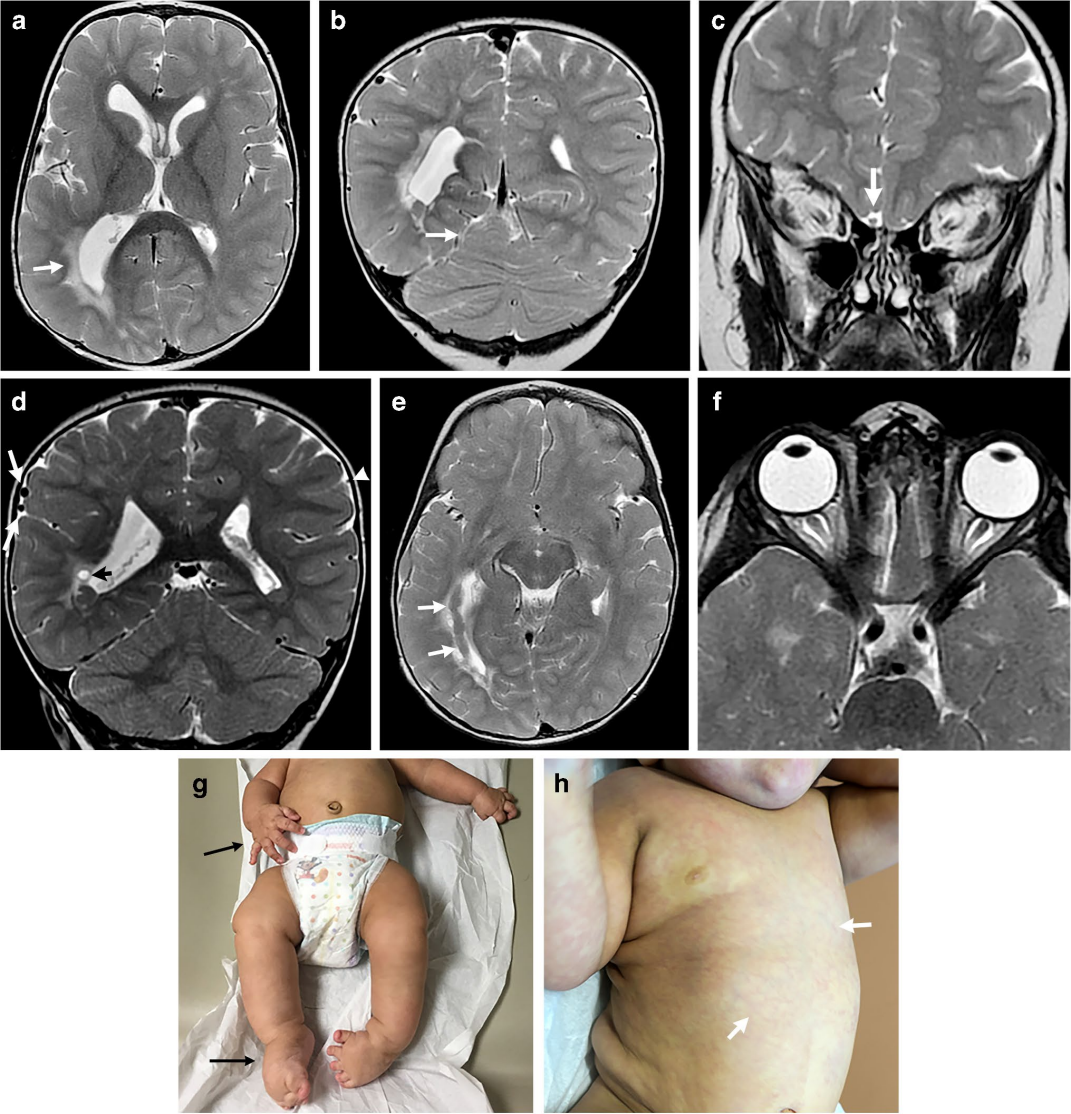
Megalencephaly-Capillary Malformation (MCAP) syndrome in a 19-month-old-boy with a genetically confirmed *PIK3CA* mutation presenting with macrocephaly, facial dysmorphism, somatic overgrowth, capillary malformations, generalized hypotonia and developmental delay. a-f Axial and coronal T2-weighted images show an enlarged right cerebral hemisphere compared to the left with associated ventricular enlargement and hyperintense signal within the periventricular white matter (a, *white arrow*; d, *black arrow*). There is enlargement of the right superior cerebellar vermis (b, *arrow*), right olfactory bulb (c, *arrow*), and right cerebral cortical veins (d, *white arrows*) compared to the normal left cortical veins (d, *arrowhead*). Subependymal heterotopia in the right temporal and occipital lobes is present (e, *arrows*). Hypertelorism (f). g A clinical photo shows enlargement of the right upper and lower extremities (*arrows*) with associated deformities and bilateral club feet. h A clinical photo shows capillary malformation in a cutis marmorata-like appearance
